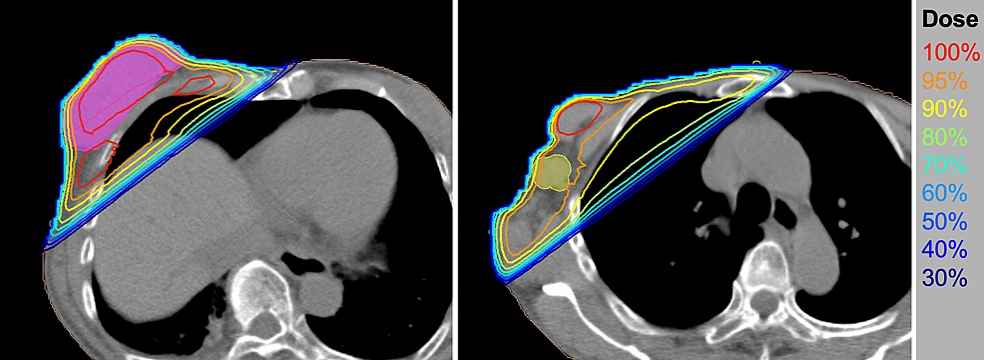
Treatment plan for radiotherapy. (A) A plane showing the primary tumor (solid magenta). (B) A plane showing the axial lymph node metastasis (solid yellow). Isodose lines are shown on computed tomography images.
